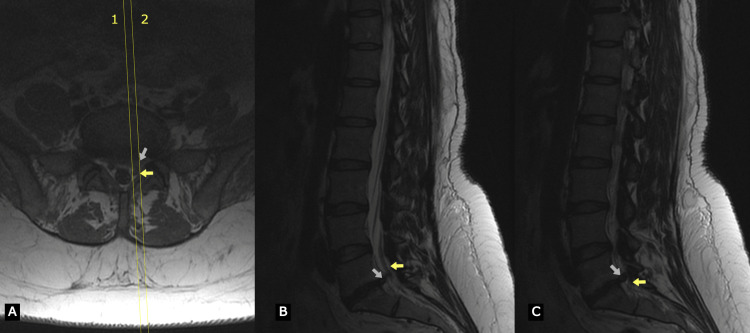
T2 weighted images of lumbar spine MRI that present a left paracentral disc herniation resulting in compression of the left S1 root Marks 1 and 2 in image A correspond to images B and C, respectively. The white arrow indicates disc herniation while the yellow arrow indicates S1 root.
